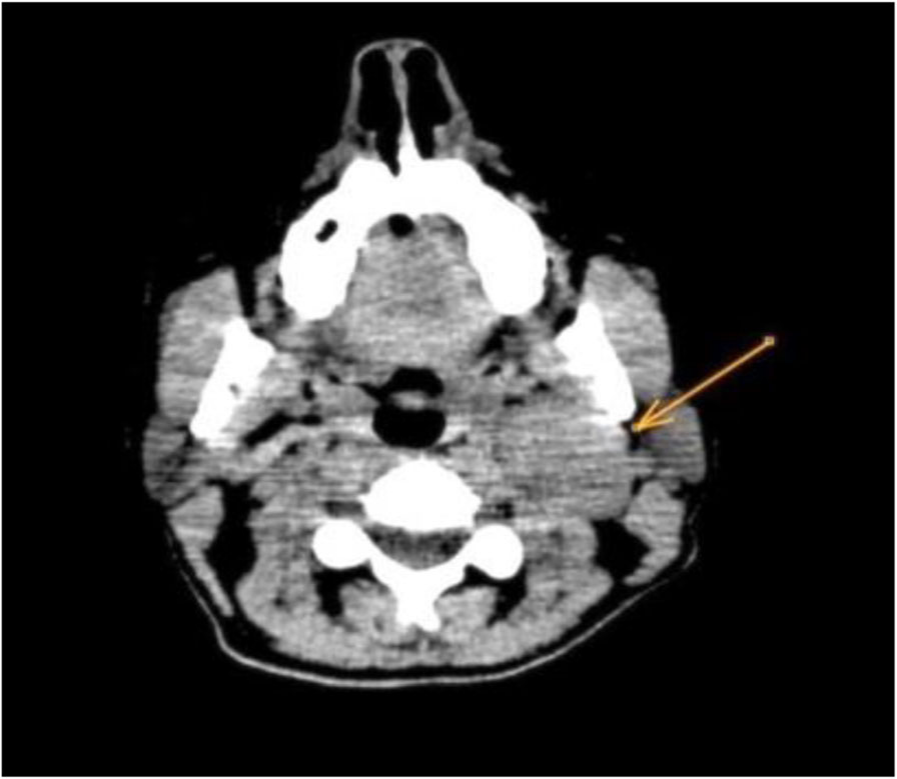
CT scan showing a left neck mass (arrow) compatible with carotid glomus
